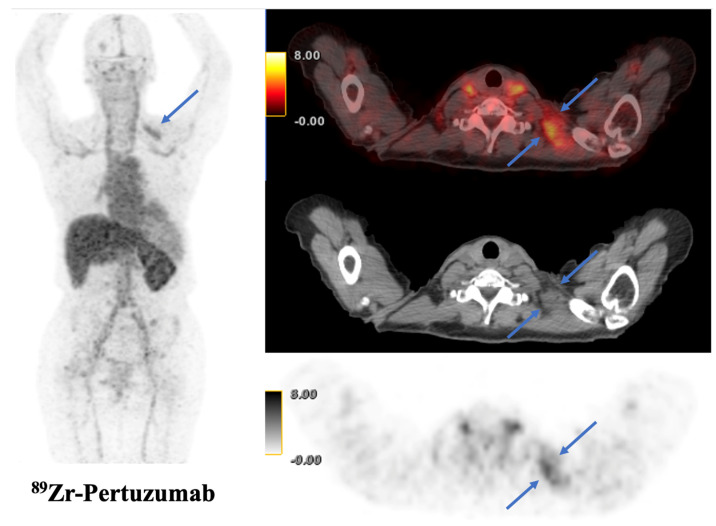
This is a 69-year-old female with left breast invasive ductal carcinoma (ER+, PR+, and HER2+) who has received several rounds of HER2-targeted therapy, including ado-trastuzumab emtansine (TDM-1), and was on gemcitabine, trastuzumab, and pertuzumab therapy at the time of ^89^Zr-pertuzumab PET/CT. Tracer avidity was visualized in a known left supraclavicular lymph node metastasis (blue arrows), with SUVmax 5.1.
